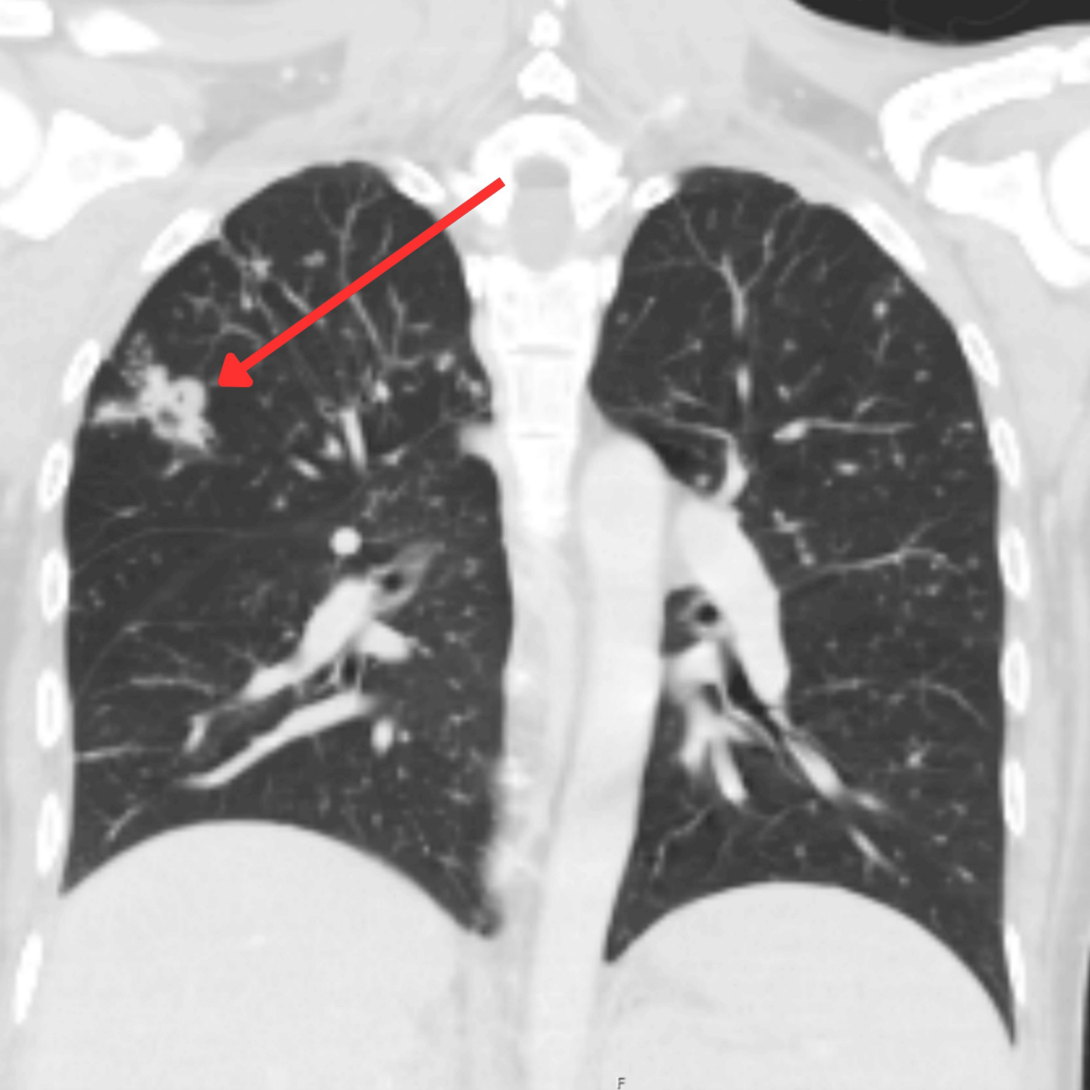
CT chest demonstrating a spiculated cavitating opacity in the right upper lobe, indicated by the red arrow.
